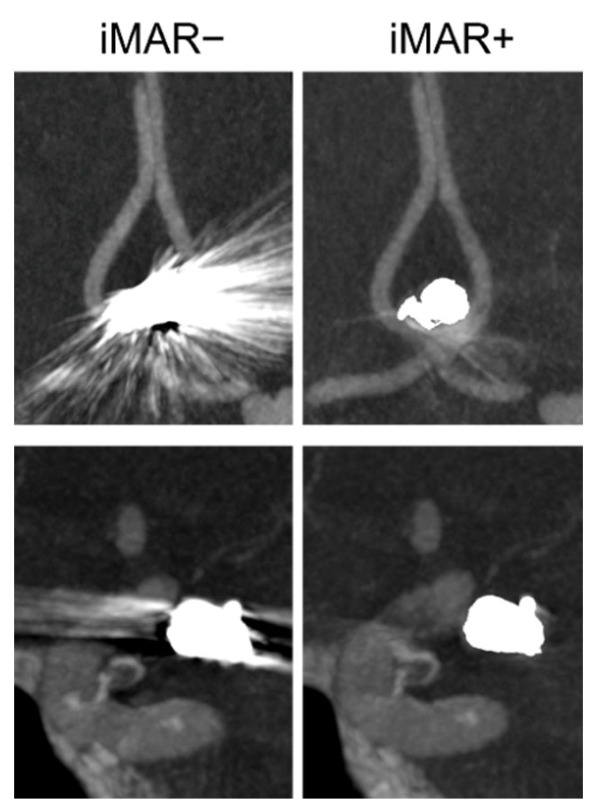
Side-by-side examples of FD-CTA images without/with application of the iMAR (iMAR−/iMAR+) in patients with treated intracranial aneurysms via endovascular coiling. Upper row shows a patient with a treated aneurysm of the anterior communicating artery, basal row shows a patient with a treated aneurysm of the internal carotid artery. In the iMAR− images of both cases, the parent vessels are not (upper case) or poorly differentiable (basal case), whereas in the iMAR+ images, the parent vessels are very well differentiable.
